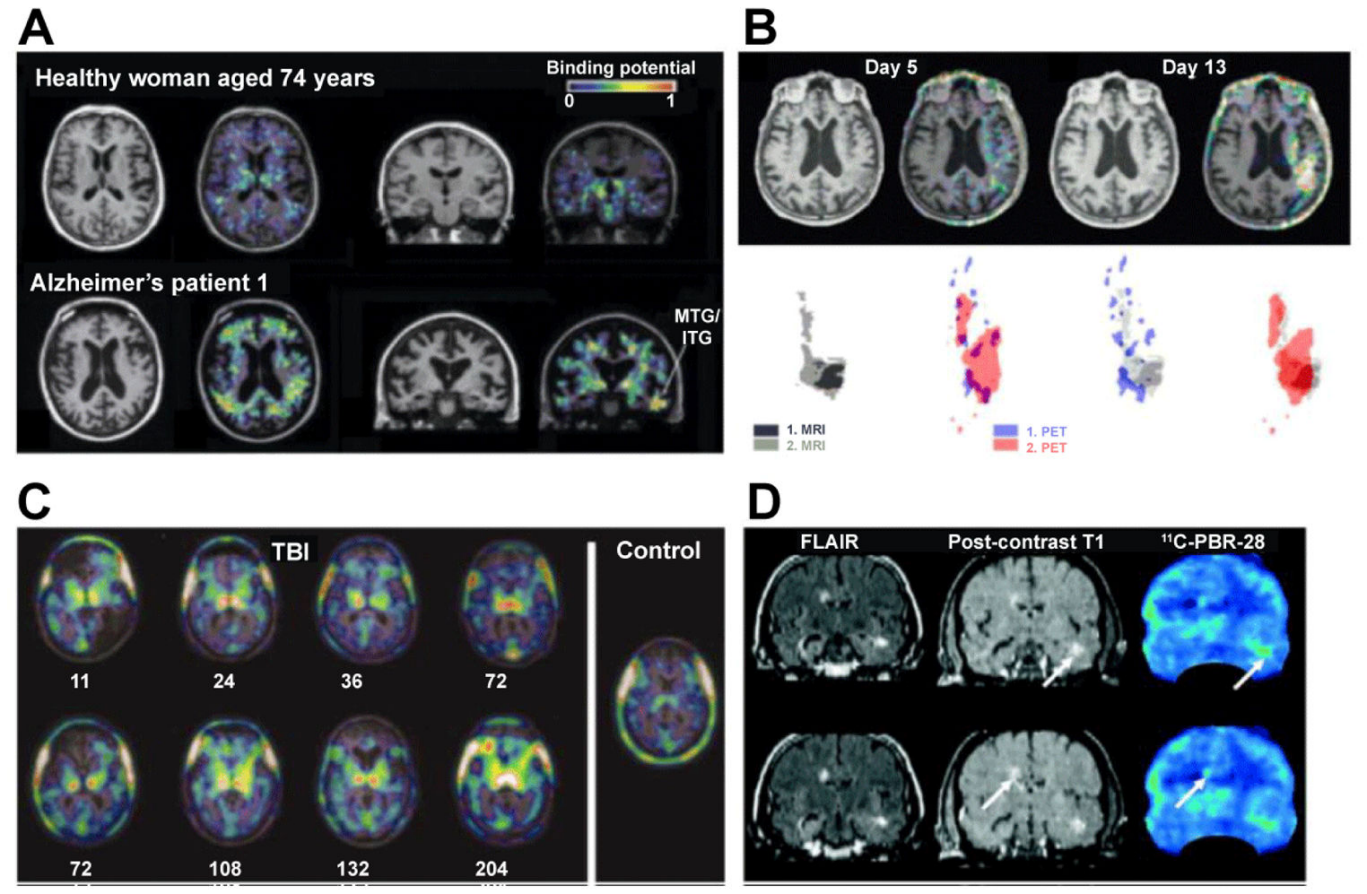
TSPO PET imaging in neuroinflammation. (A) 11C-PK11195 PET in patients with Alzheimer’s disease shows increased uptake suggestive of microglia activation in the entorhinal, temporoparietal and cingulate cortex. (B) ^11^C-PK11195 PET in a patient with acute ischemic stroke. Coregistered T1-weighted MRI and PET demonstrated ^11^C-PK11195 enhancement surrounding the infarct seen on MRI on day 5, while considerable overlap between MRI and PET was found on day 13. (C) Chronic microglia activation following TBI as evaluated by ^11^C-PK11195 PET, where increased uptake in the thalami of all TBI subjects is seen compared to control individuals. (D) In a patient with MS, focally increased uptake of the TSPO PET agent ^11^C-PBR28 is seen in gadolinium enhancing and FLAIR hyperintense MRI lesions (arrows). (Modified from Cagnin et al. [90] Gerhard et al. [93], Ramlackhansingh et al. [92], and Oh et al. [124] with permission.)
